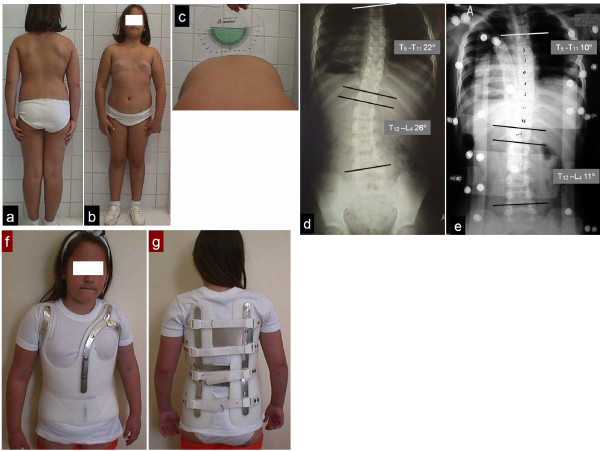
Figure 9a front and 9b back view of a pre-menarche scoliotic child, 8 years of age with Idiopathic Scoliosis, 9c scoliometer reading 7 degrees, 9d radiographic study: double curve, Risser 0, T5 - T11: 22 degrees Cobb angle, T12 - L4: 26 degrees Cobb angle, 9e immediate post brace application correction, T4 - T11: 10 degrees Cobb angle (54,5% correction), T12 - L4: 11 degrees Cobb angle (57,7% correction), 9f anterior and 9g posterior view of the girl with the DDB on, a double major curve module with two derotation blades, (thoracic and lumbar).
